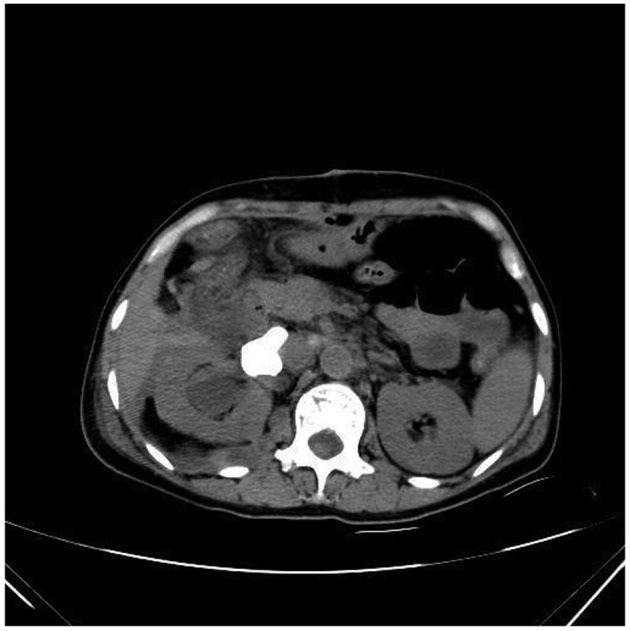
Plain and enhanced computed tomography scan of the liver showing a slight reduction in volume and cavernous hemangioma in the right posterior liver.
